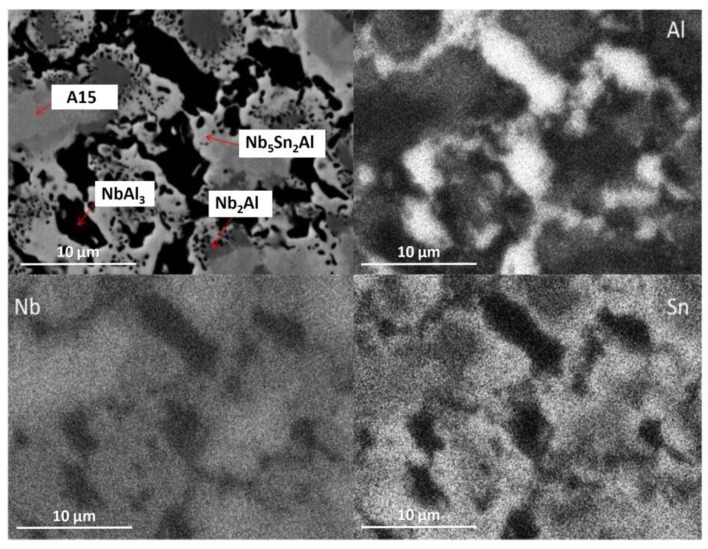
BSE image and X-ray element maps of IP5-HT 900 °C/100 h.
